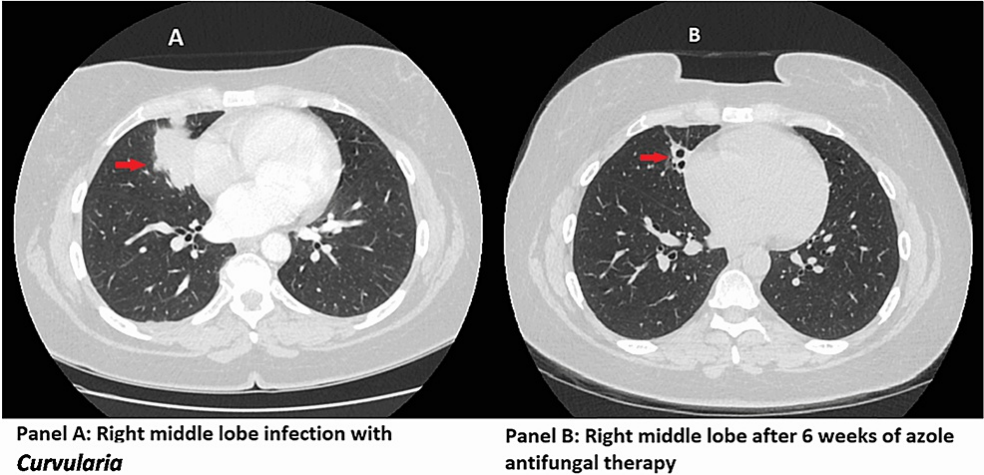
CT chest imaging CT: computed tomography
